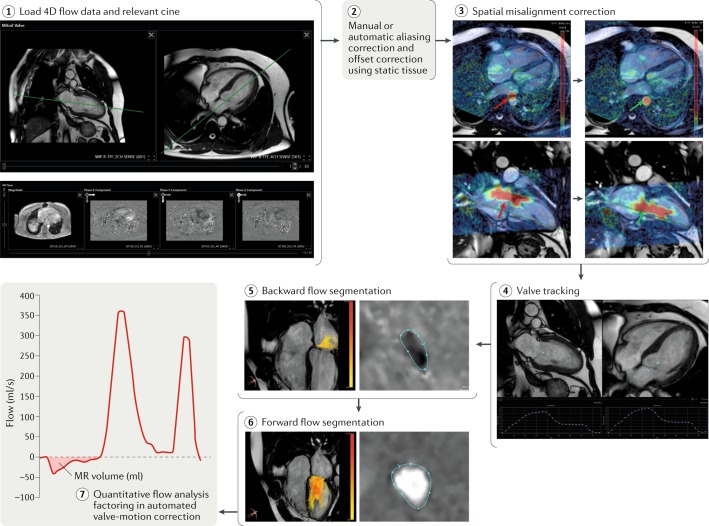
4D-flow cardiovascular magnetic resonance imaging analysis. Step 1: load the two-chamber and four-chamber cardiovascular magnetic resonance imaging cines (upper panel) and 4D phase-contrast flow and 3D phase-contrast data (lower panel). Step 2: depending on the software tool, perform manual or automated aliasing and velocity offset corrections with the use of the static background tissue. Step 3: an attempt to check for spatial misalignment between the cines and the 4D-flow data should be made because the presence of any major misalignment between them will introduce errors in the quantification of valvular flow. The red arrows indicate the spatial misalignment of both the descending aortic flow (in systole) and the mitral inflow (in diastole). The green arrows indicate how this misalignment has been corrected. Step 4: on both the two-chamber and the four-chamber cines, place two landmark points to mark the valve plane. This marking should be done for the complete cardiac cycle. Software solutions (for example, CAAS software, PIE Medical Imaging, Netherlands) can now automatically track the valve over the complete cardiac cycle. After ensuring the valve is properly tracked, generate a phase-contrast, valvular reformatted plane. Steps 5–6: on the valvular reformatted plane, segment the mitral regurgitant backward flow during left ventricular systole and the forward flow during diastole. The regurgitant plane should be perpendicular to the regurgitant jet. If multiple jets exist, one should make an attempt to evaluate each of them to quantify the total mitral regurgitation (MR). Step 7: quantify valvular forward and backward flow after accounting for the through-plane motion of the valve plane. Similar steps can be used to quantify aortic, tricuspid and pulmonary valvular flows with the use of the 4D-flow data set.
